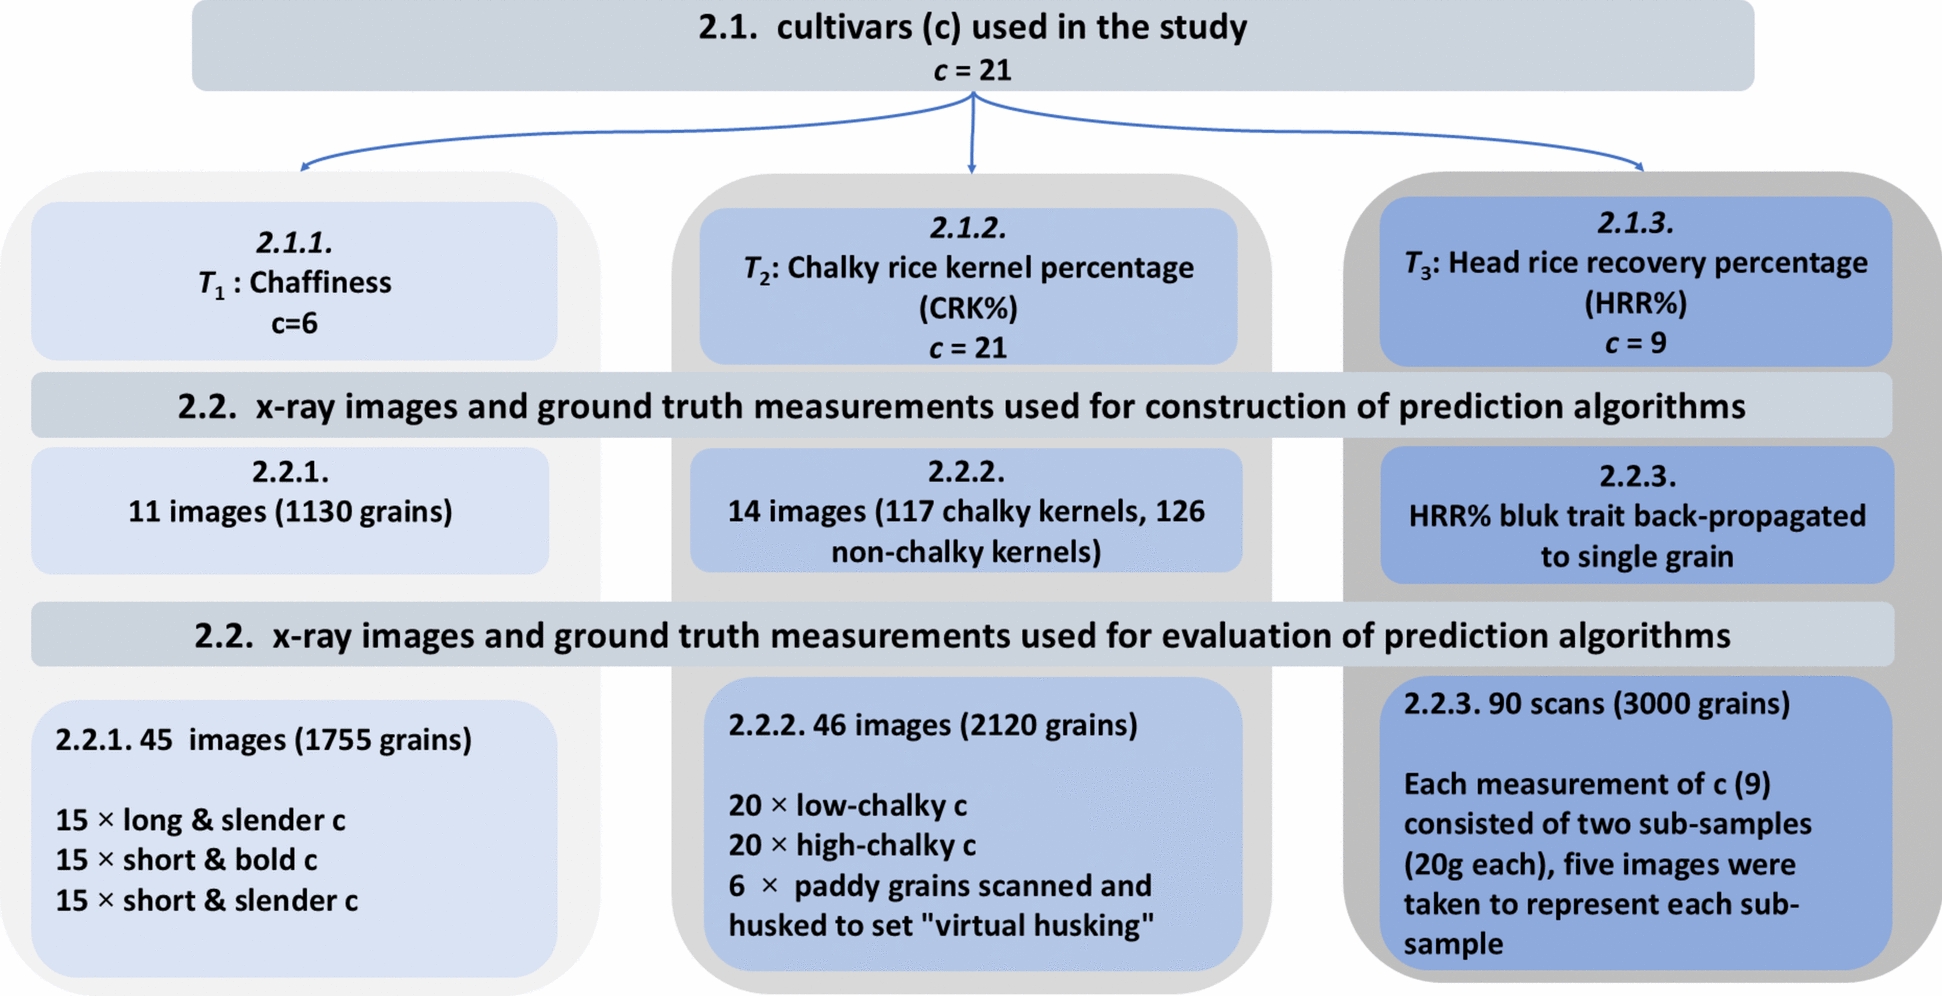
Overview of numbers of cultivars (c), X-ray images and grains used for the development of trait prediction algorithms for the three traits (T): T_1_ chaffiness (2.1.1), T_2_ chalky rice kernel % (CRK%, 2.1.2) and T_3_ head rice recovery % (HRR%,2.1.3)
